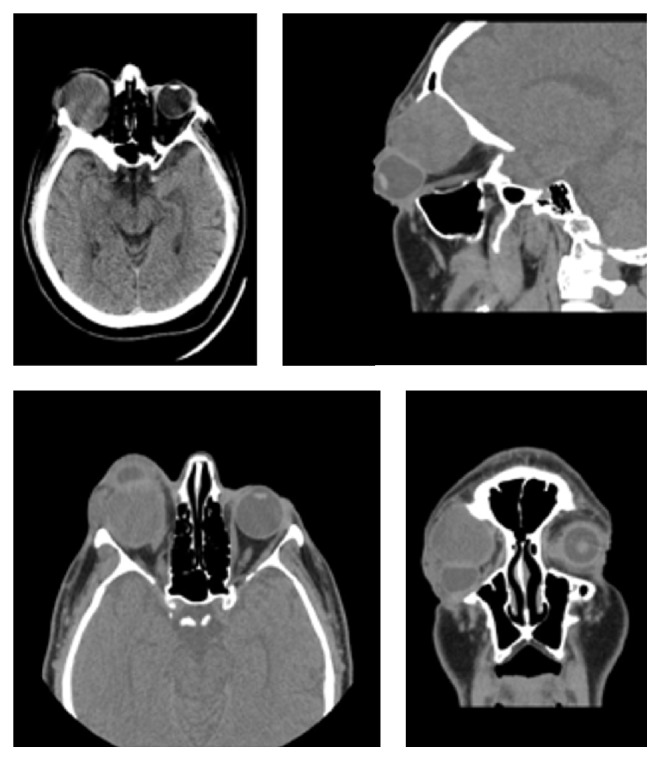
CT axial, sagittal, and coronal views of cyst causing proptosis.
